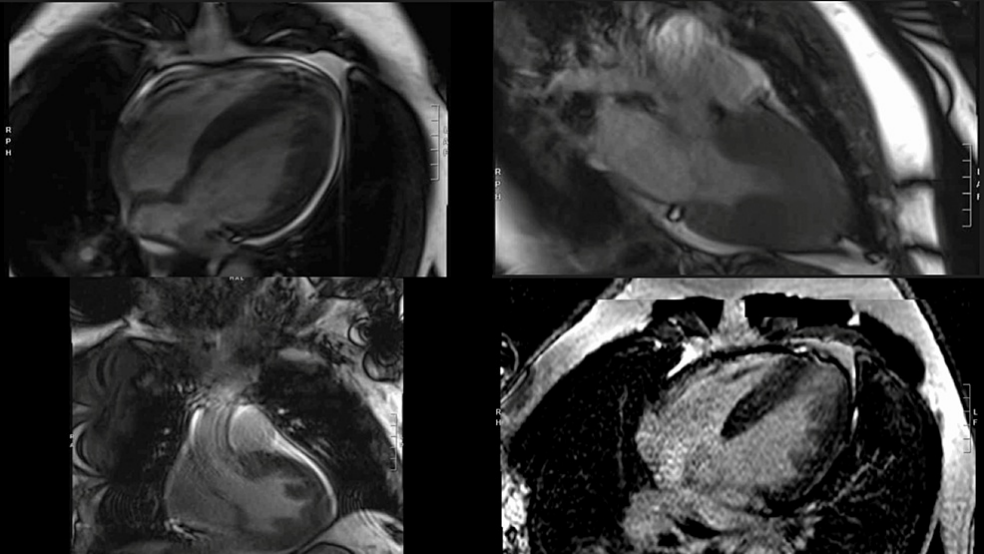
Cardiac magnetic resonance imaging with and without contrast.
